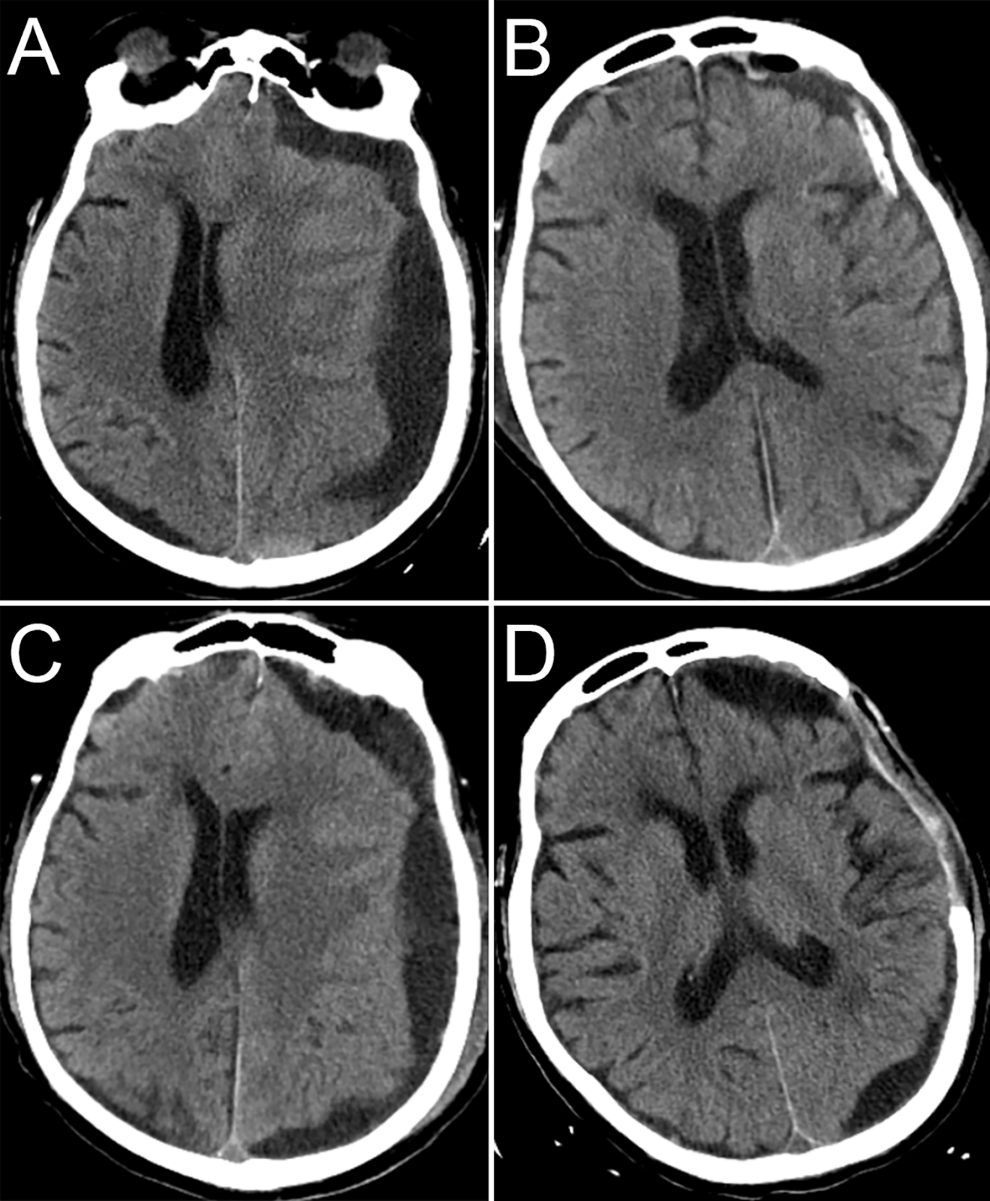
CT images during hospitalization. CT before the first surgery (A), CT after the first surgery (B), CT before the second surgery (C), CT 1 month after the second surgery (D).
